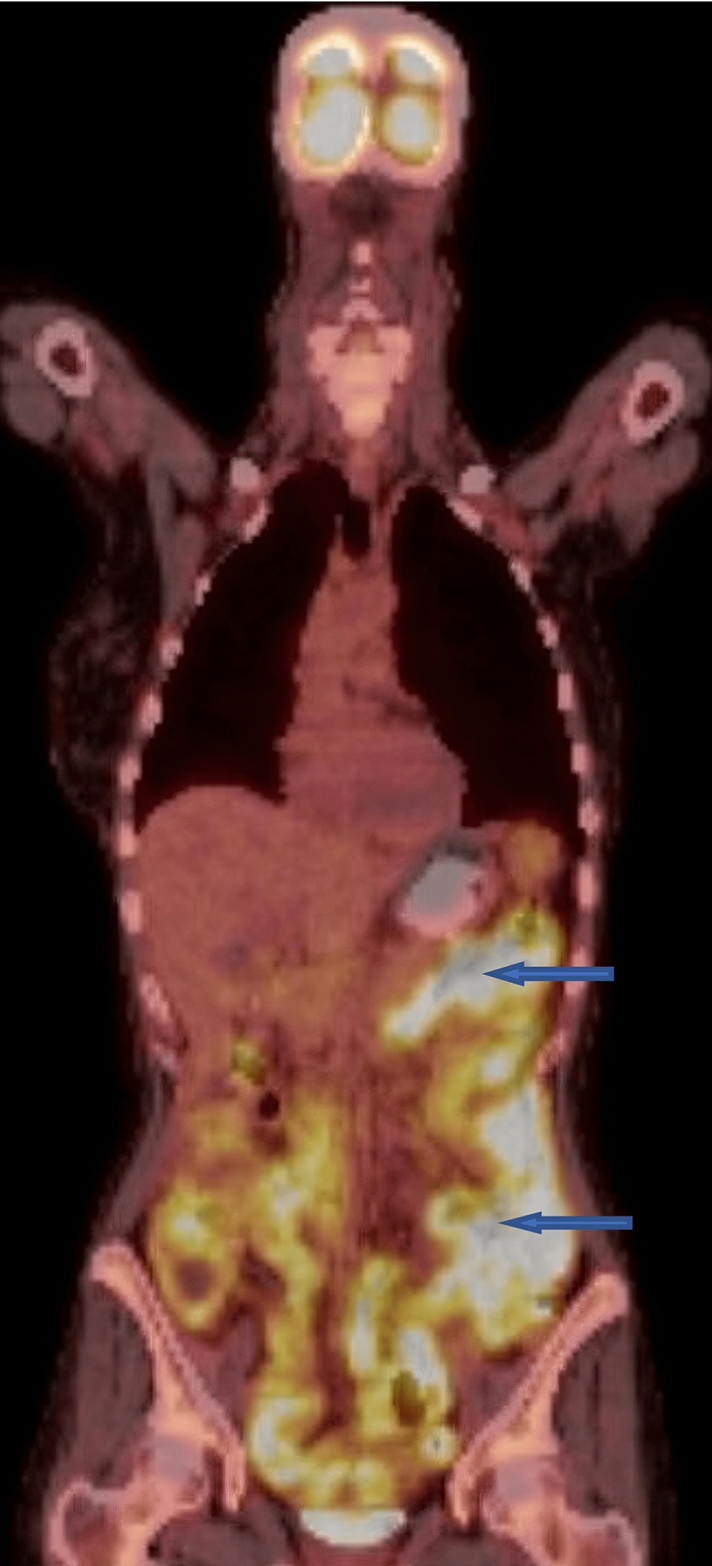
The FDG PET scan reveals diffuse intense FDG avidity (blue arrows) throughout much of the small and large bowel FDG: Fluorodeoxyglucose, PET: Positron emission tomography
